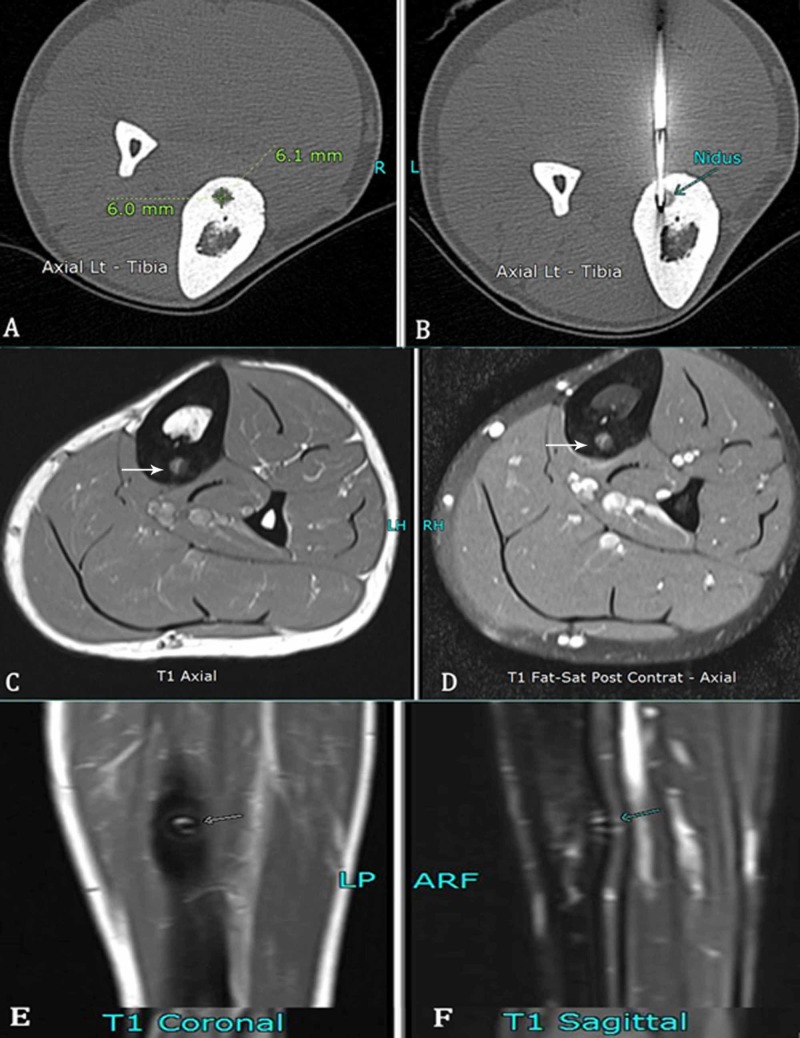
Various imaging findings of a 24-year-old male with an osteoid osteoma in the left tibia. A) Unenhanced CT axial section showing osteoid osteoma in the tibia measuring 6.1 x 6 mm. B) Soloist needle in the nidus (arrow). C-F) Follow-up MRI after six months shows non-enhancing nidus (arrows), representing successful ablation.
